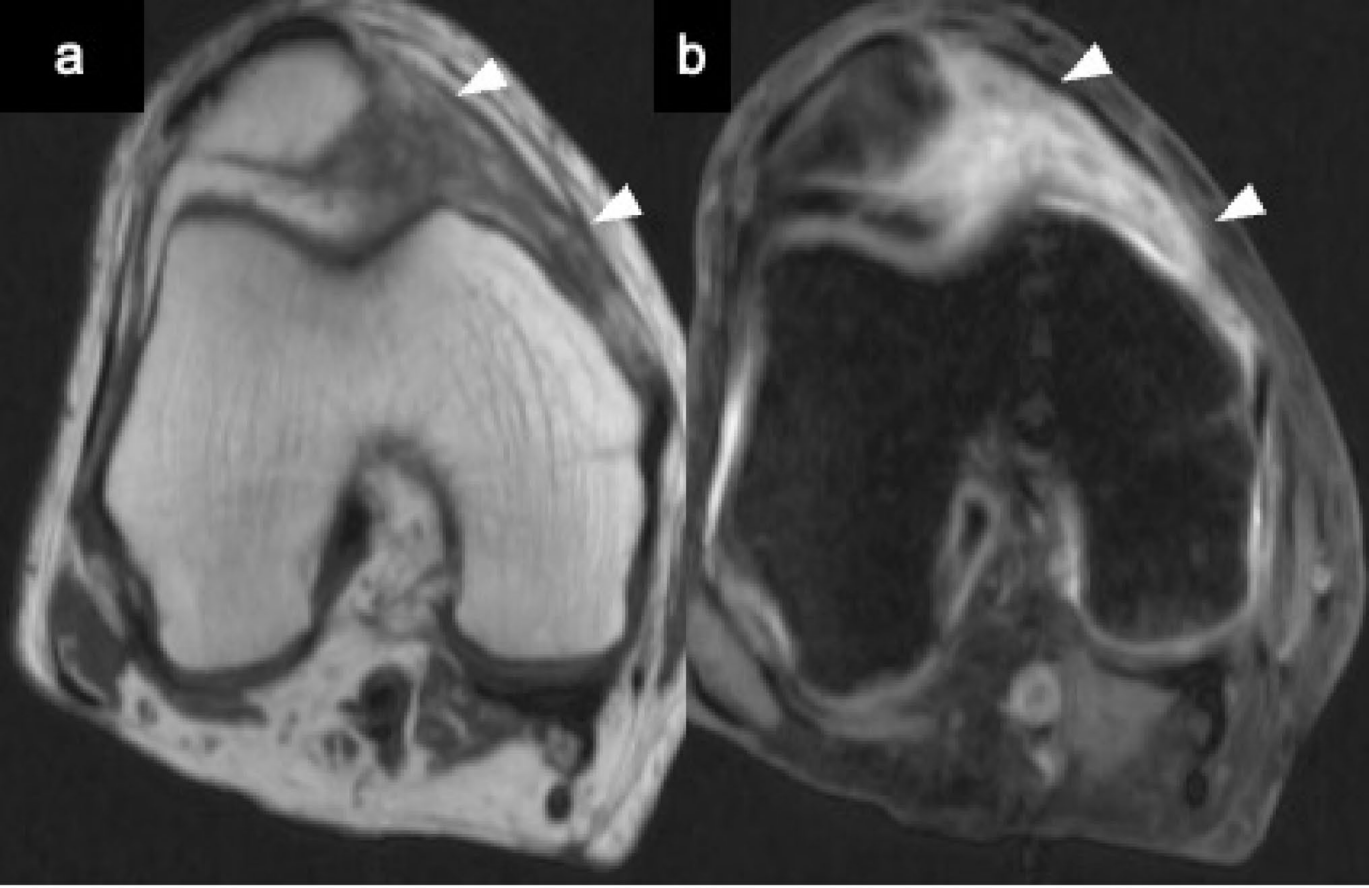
Metastasis from salivary gland cancer. Axial T1-weighted MR image (a) shows an ill-defined soft tissue mass (arrowheads) anterior to the lateral condyle of the femur. Marked enhancement is seen on fat-suppressed T1-weighted MR image obtained after contrast administration (b)
